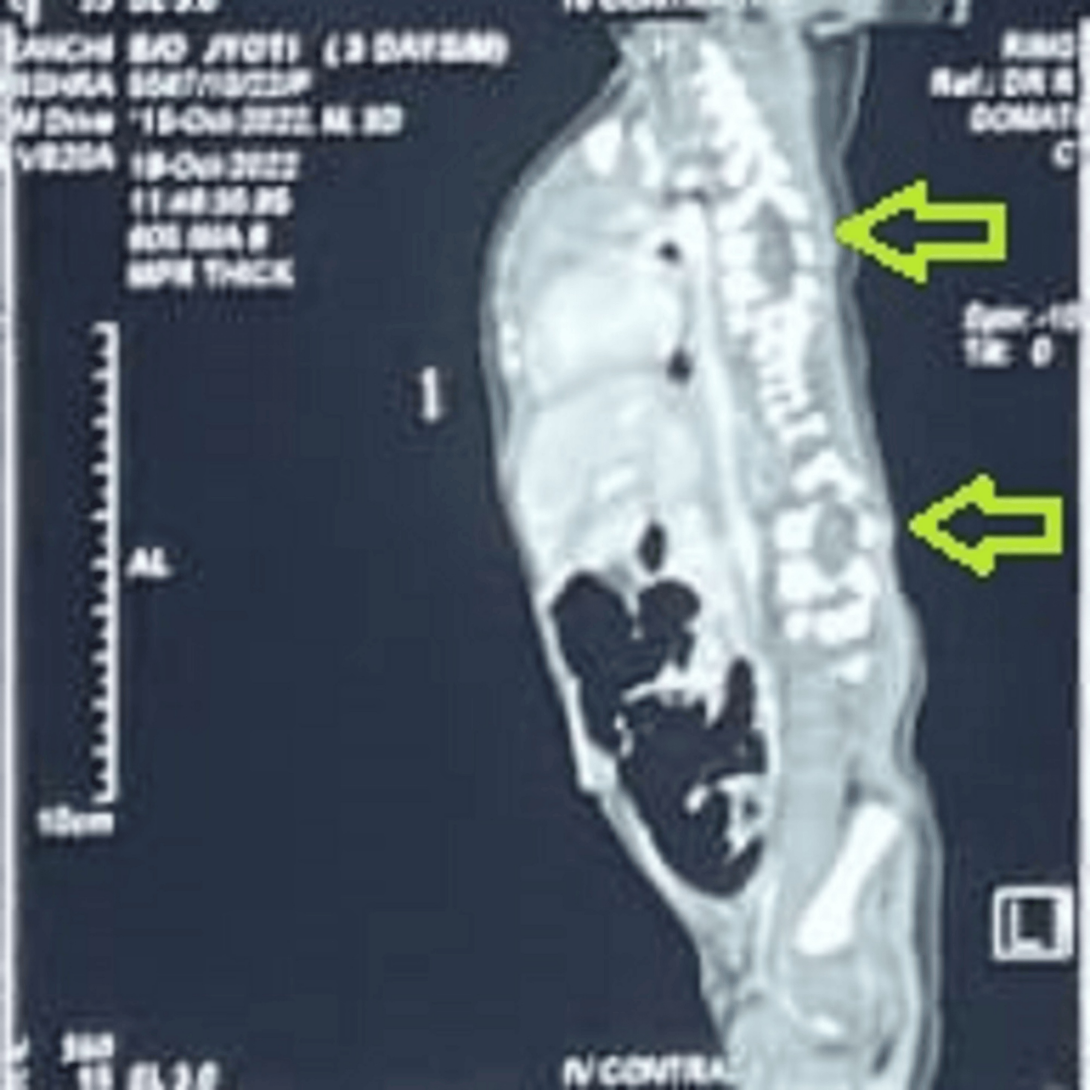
Computed tomography of the thorax and abdomen of a neonate with spondylo-thoracic dysplasia. Shows ground-glass opacities in bilateral lung fields with a right mediastinal shift, hemivertebrae of D6, D7, and D8, a bifid 8th vertebral head, an absent left 8th rib, and L4-S5 spina bifida.
